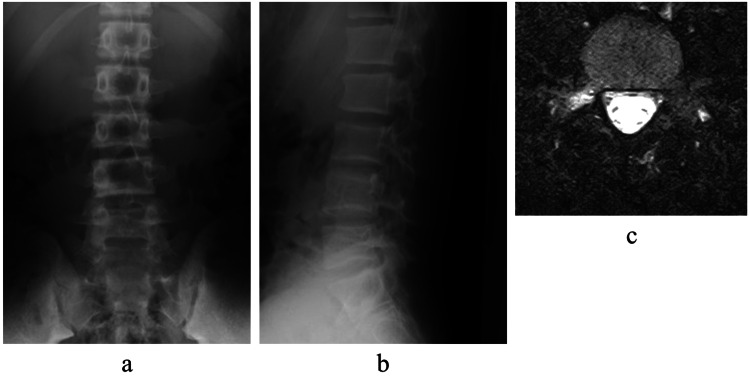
Plain radiographs and magnetic resonance images at first examination Plain radiographs at the first examination showed no obvious abnormalities (a, b). Short tau inversion recovery magnetic resonance images (STIR-MRI) showed high signal change in the right pedicle of L5 (c).
